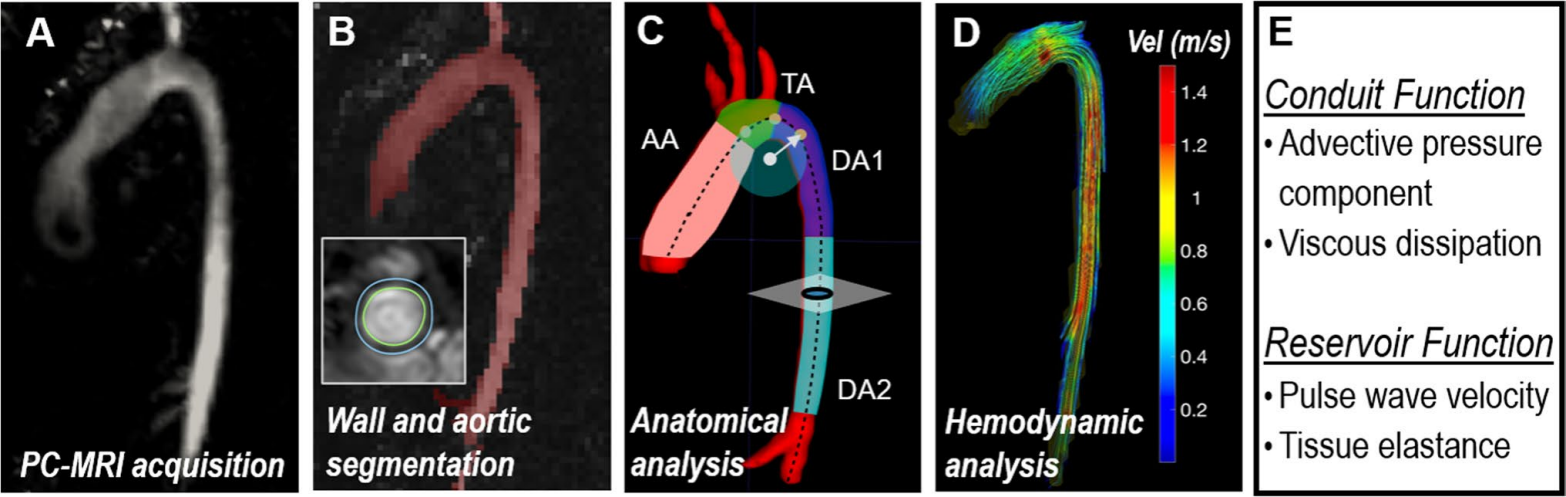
Methodological overview to extract the conduit and reservoir functions of a vascular segment. A Acquisition of 4D flow MRI data. B Anatomical segmentation from images of peak systolic velocity magnitude. C Subdivision of the aorta into 4 segments and extraction of shape metrics (centreline, curvature, diameter). D Flow velocity vectors and magnitude iso-contours reconstructed from PC-MRI data. E Extraction of descriptors of conduit and reservoir function
